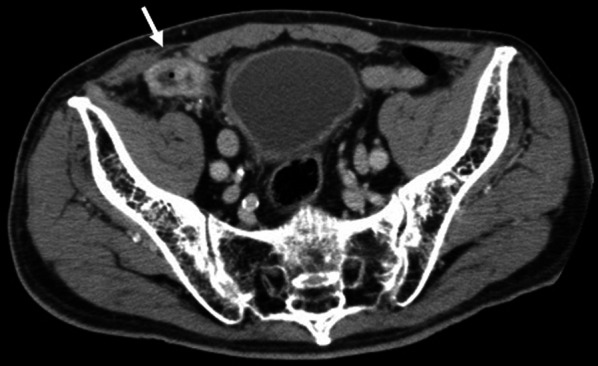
Computed tomography (CT) findings upon admission. The CT scan demonstrates a peri-appendicular abscess (arrow)
